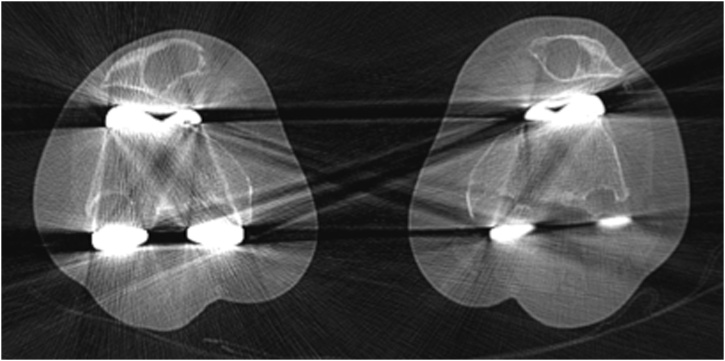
Computed tomography slices of both knees (left is right and right is left) at the level of the femoral metaphyses. Severe bone loss is visible at the posterior aspect of the femur and patella.
